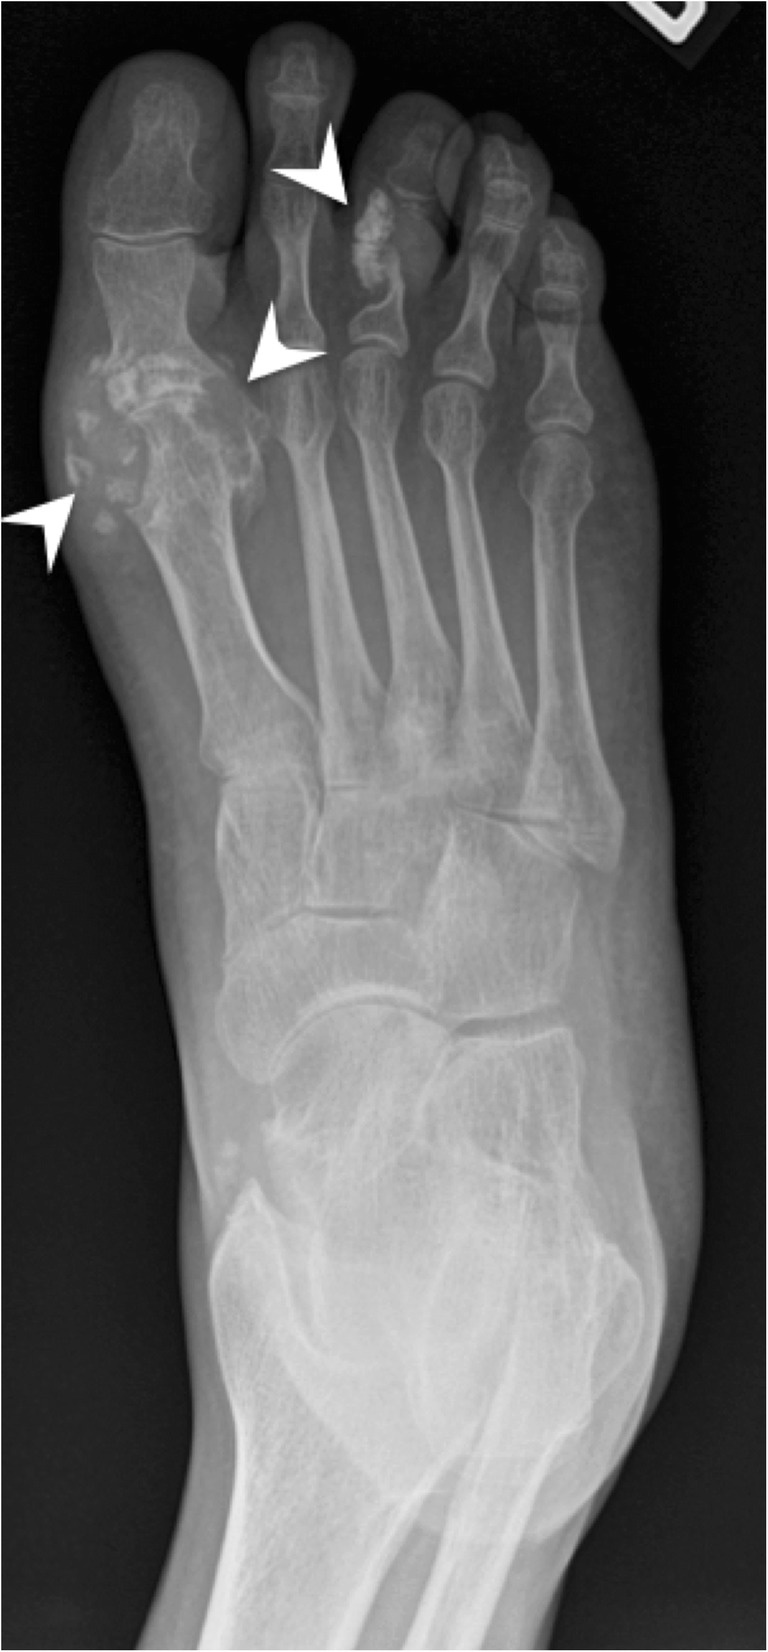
Gout. Frontal radiograph of the right foot shows tophi and erosions. Some tophi may present with mineralisation (arrowheads)
